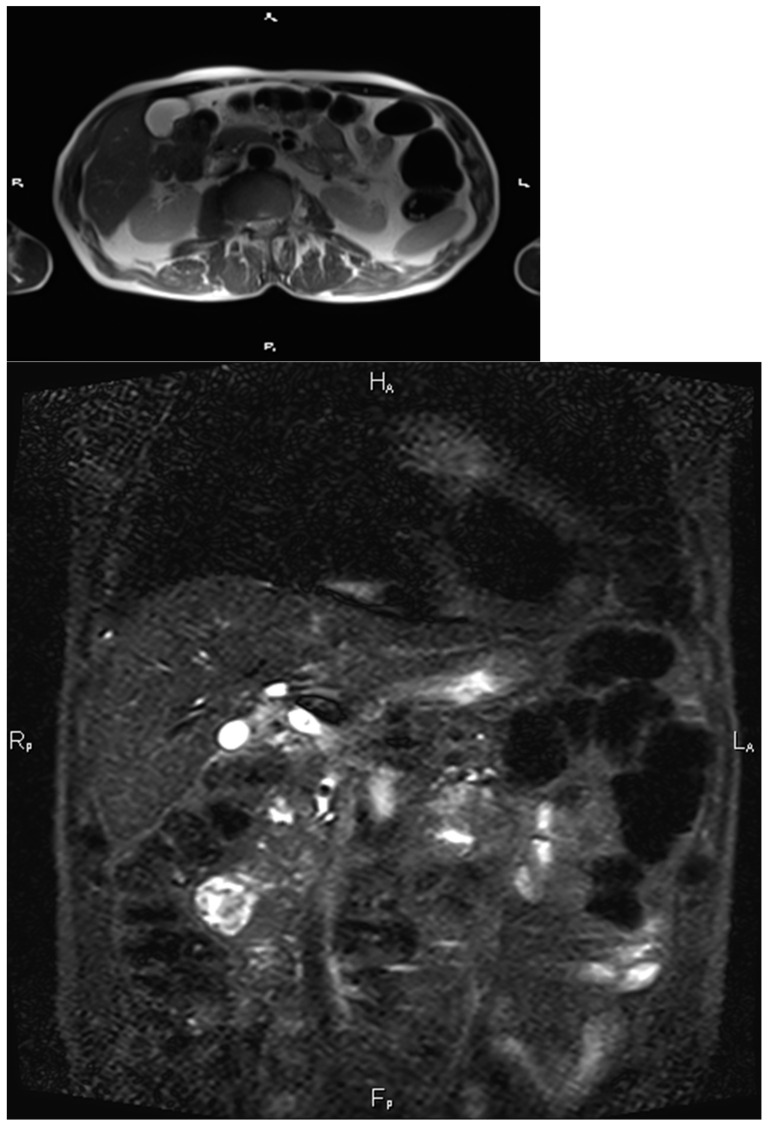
Magnetic resonance imaging of choledocholithiasis. Case courtesy of Mohammadtaghi Niknejad. From https://radiopaedia.org/?lang=us (accessed on 21 February 2023).
